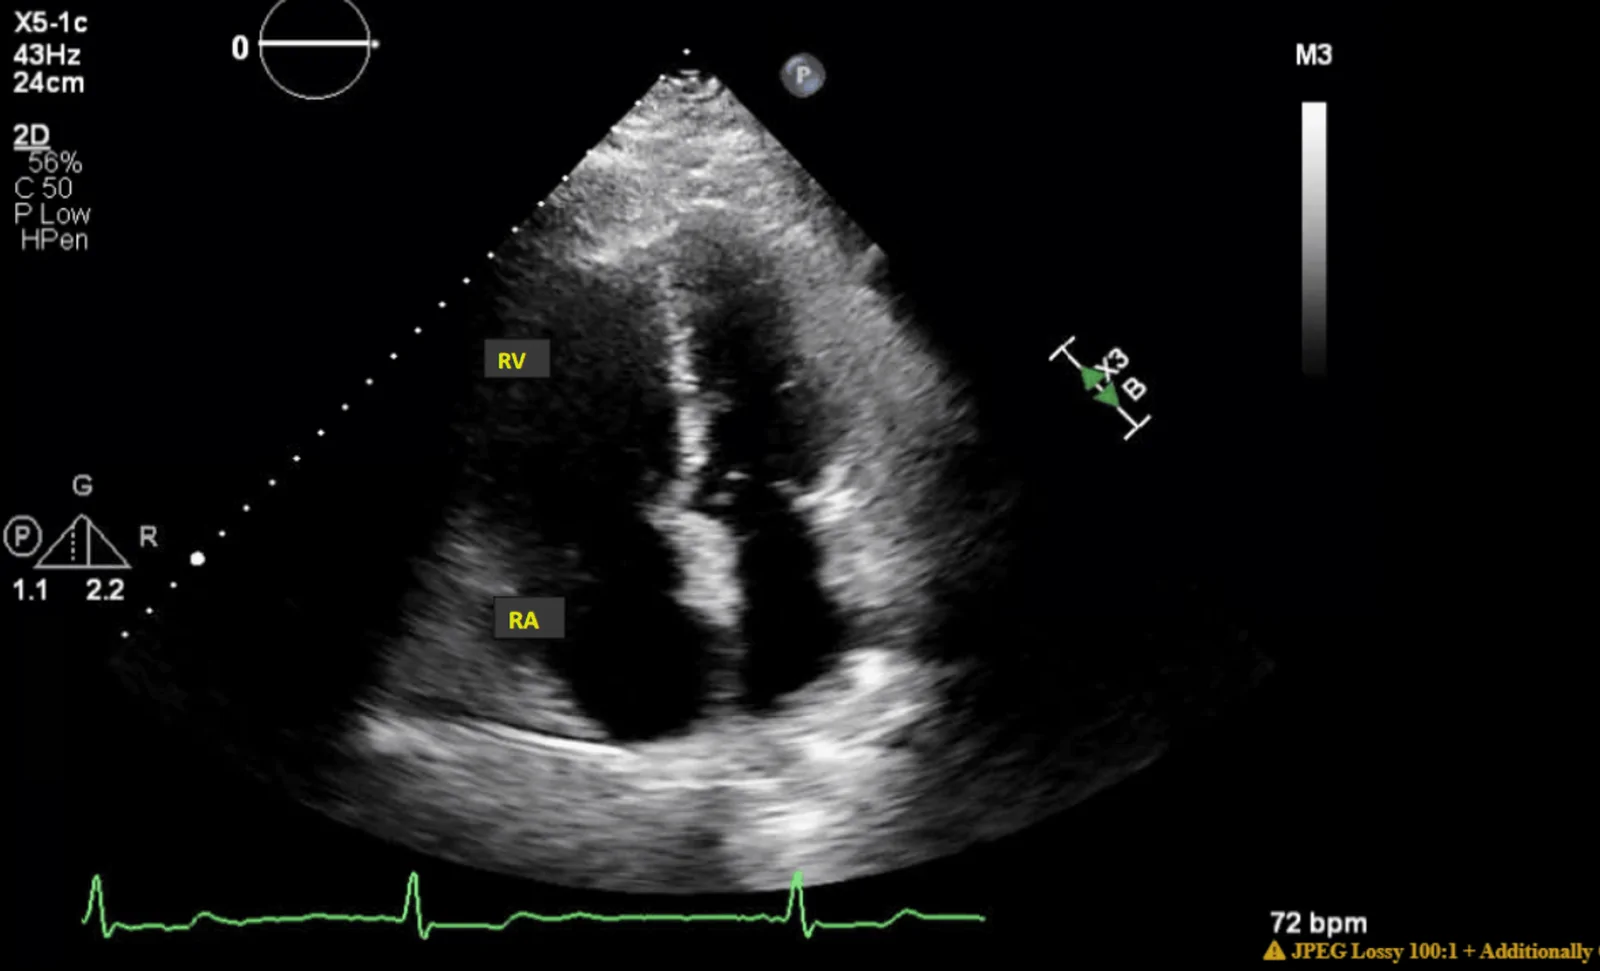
Apical four-chamber echocardiographic view demonstrating right heart chamber enlargement Apical four-chamber view demonstrating marked enlargement of the right ventricle (RV) and right atrium (RA), consistent with right heart chamber dilatation.
